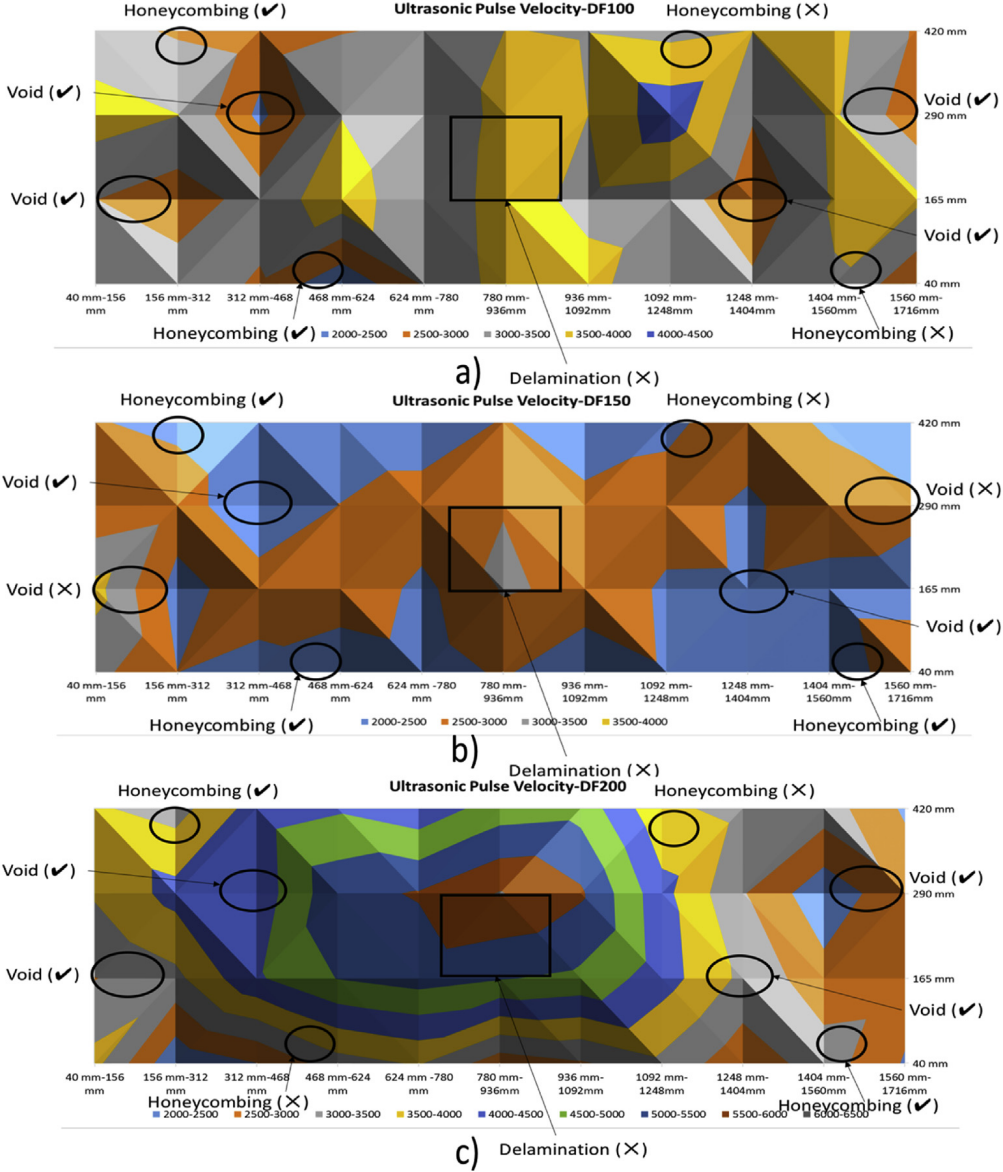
Ultrasonic Pulse Velocity-slabs with Defects - a) 100 mm, b)150 mm, and c) 200 mm.
